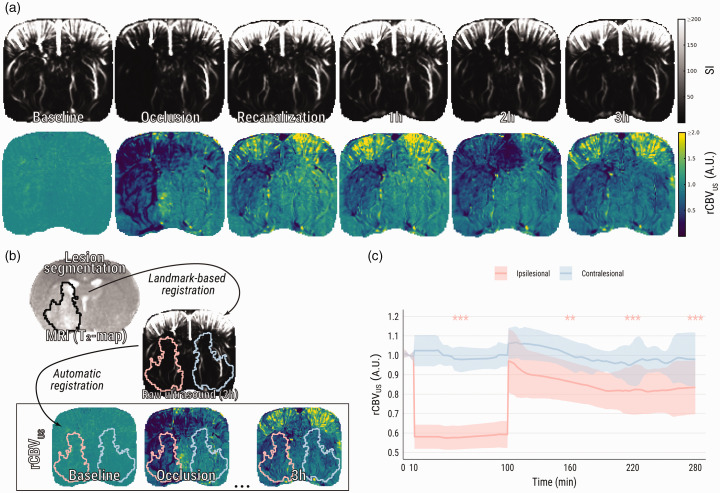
Ultrasensitive Doppler shows loss and subsequent incomplete restoration of CBV after tMCAO. (a) Representative images of raw ultrasensitive Doppler signal and relative CBV (rCBV_US_) over the course of an experiment. (b) Visual representation of the registration pipeline: MR images were co-registered with the final ultrasound images, while ultrasound images were automatically co-registered to one another. This pipeline facilitated alignment of vasogenic lesion masks from MRI (black outline on T_2_-map) to every previously recorded ultrasound image and (c) Group averages of relative CBV from ipsi- and contralesional ROIs. Asterisks indicate significant difference of ipsilesional relative CBV with respective to baseline. SI: signal intensity. *p* < .01; p < *.001.
